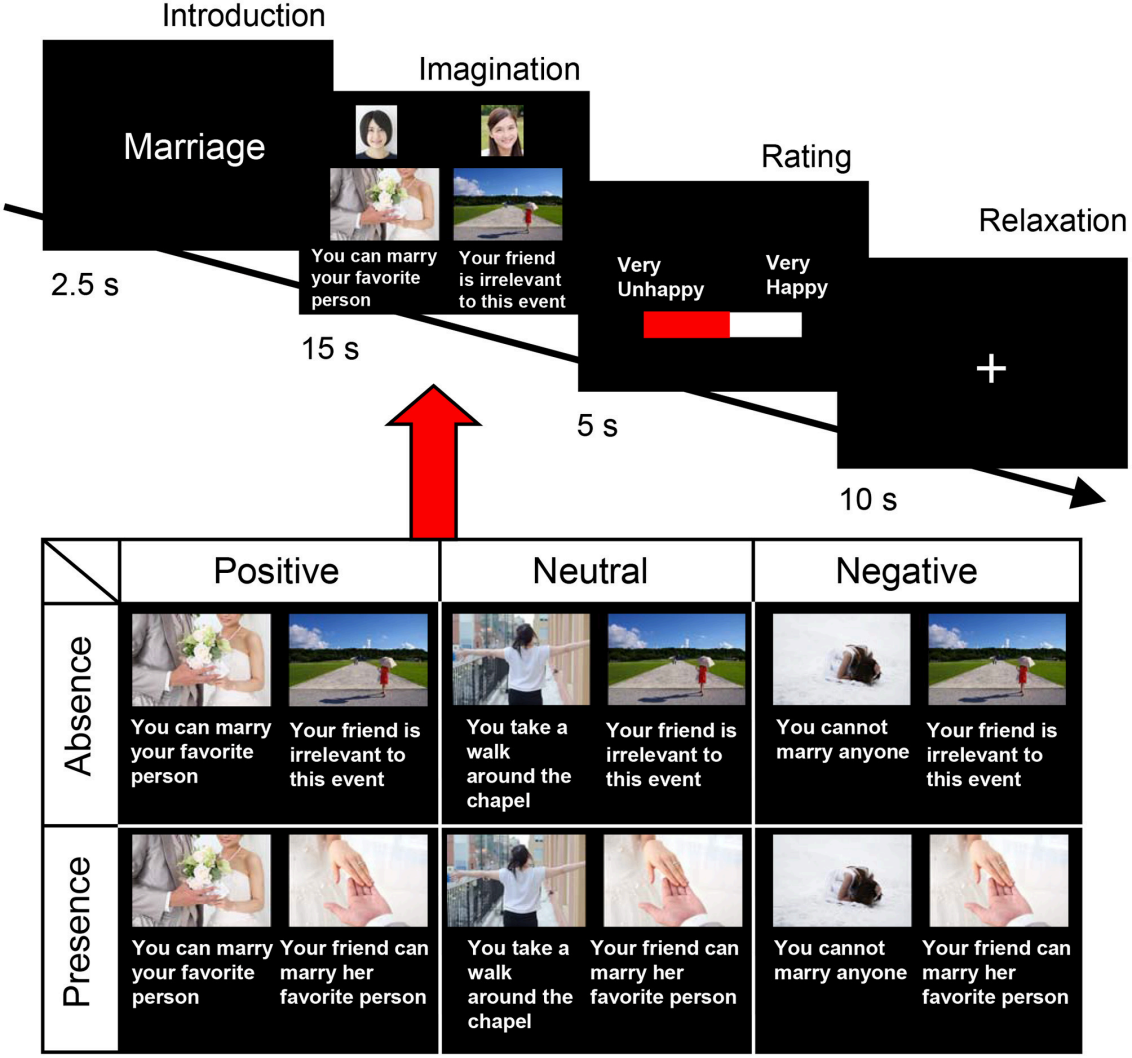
Sequence of events in each trial. Each trial consisted of the following sequence: (1) an introductory text phrase explaining the life event (2.5 s), (2) the imagination phase (15 s), (3) a rating phase (5 s), and (4) a relaxation phase (10 s). During the imagination phase, a picture was presented along with a statement pertaining to the outcome (positive/absence, neutral/absence, negative/absence, positive/presence, neutral/presence, or negative/presence). The example pictures representing each life event were actually used in the functional magnetic resonance imaging experiment in the present study. The photographs of participants were presented above the picture of each life event. Statements were written in Japanese for this task. We obtained copyright-free pictures representing each life event, as well as the sample face images used in this figure, from the Internet (http://www.photo-ac.com/).
